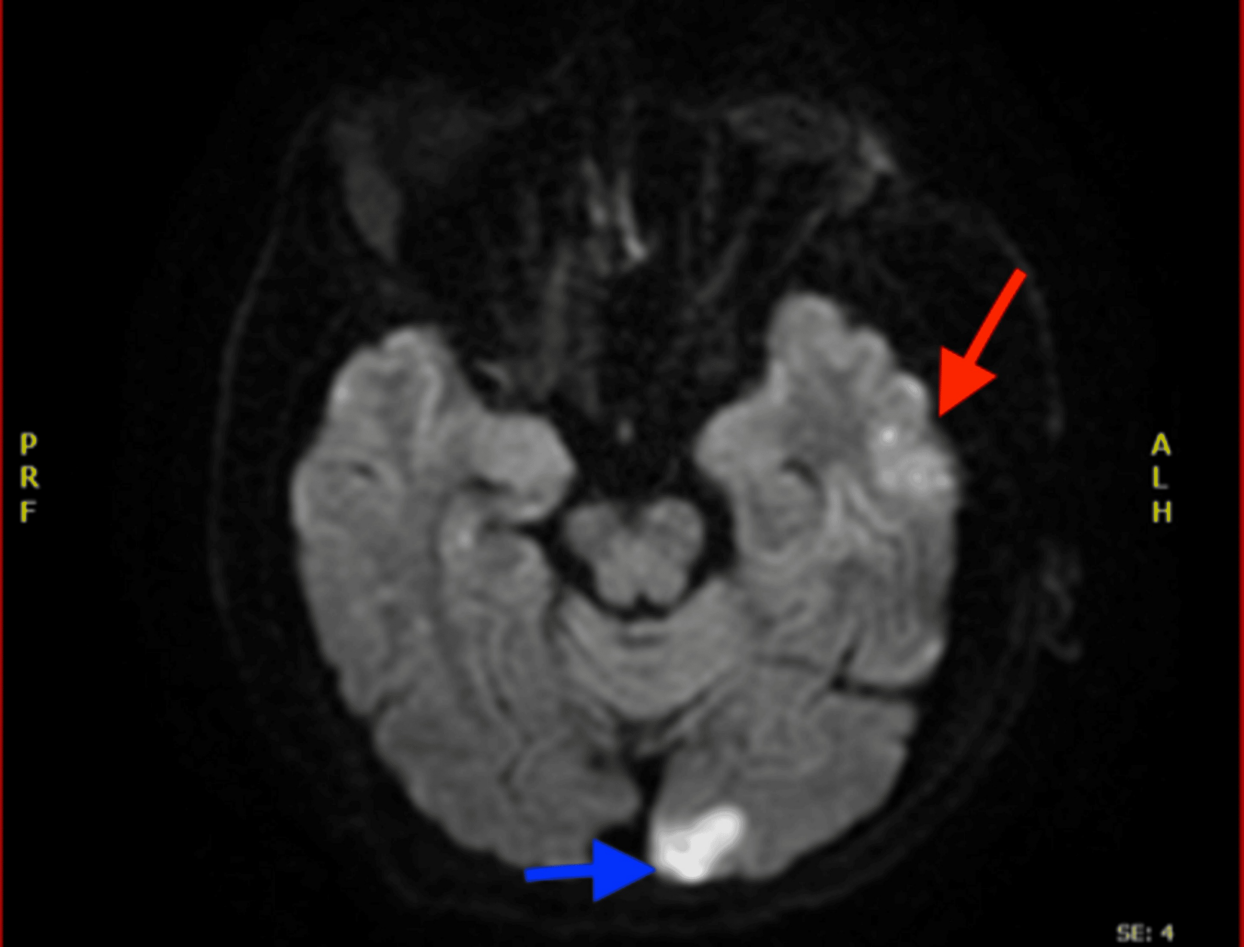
MRI of the brain showing T2 FLAIR high signal intensities in the left occipital lobe (blue arrow) and left parietal lobe (red arrow), suggestive of showering emboli
